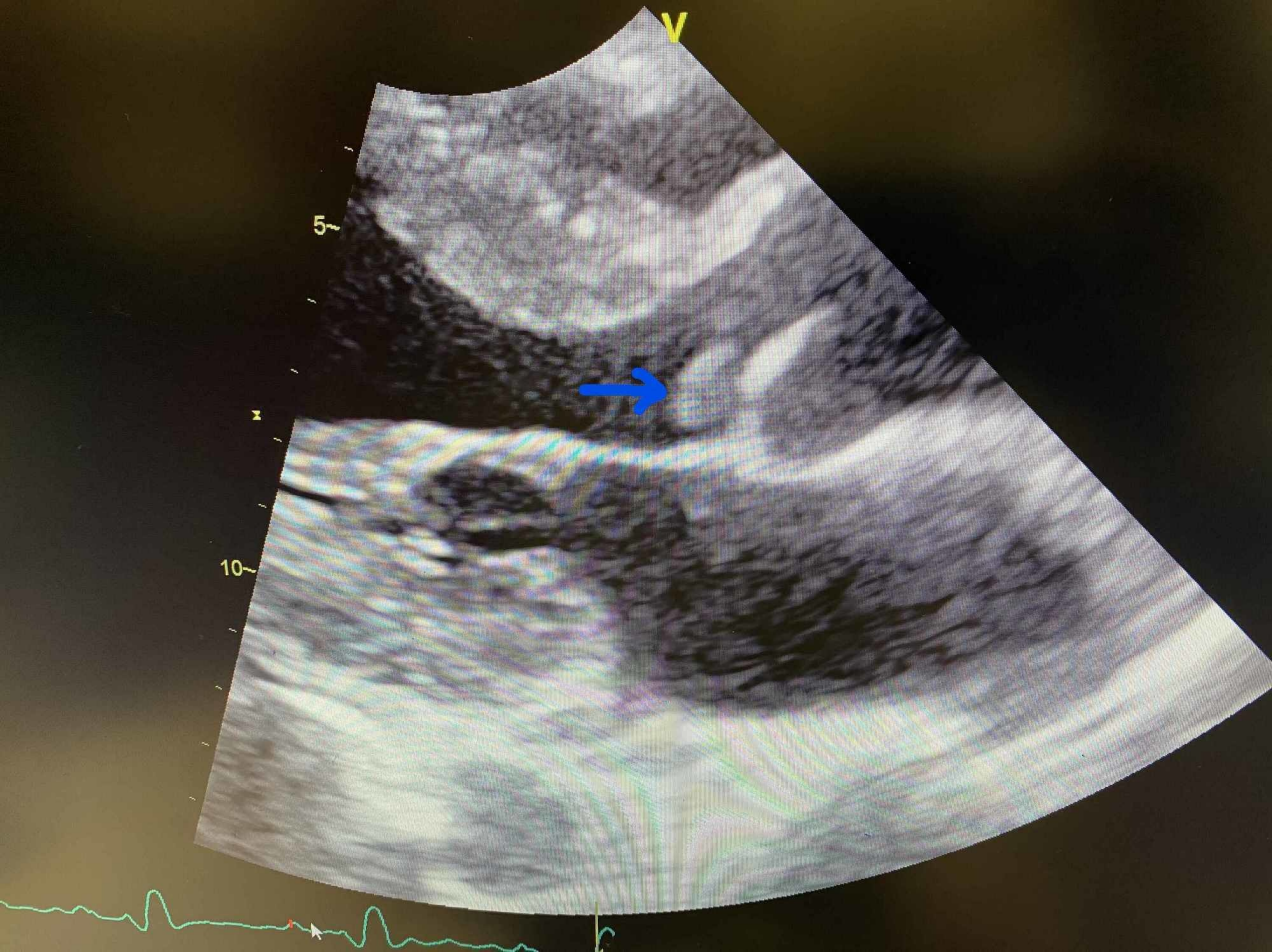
Echocardiogram showing a 1.3 x 0.7 cm aortic valve vegetation
